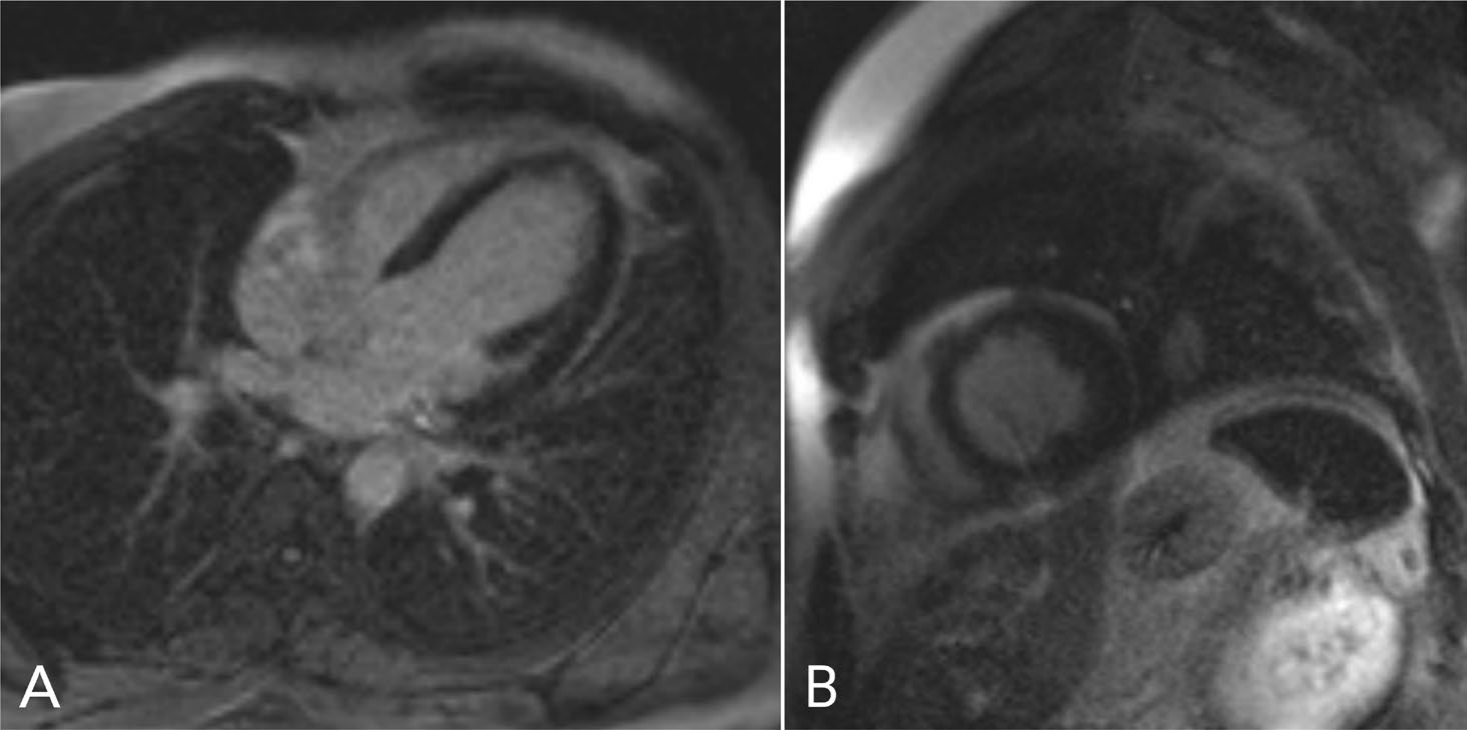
Cardiac magnetic resonance imaging: (A, B) Late gadolinium enhancement images midlevel short axis and four‐chambers show no significant myocardial infarction or fibrosis.
